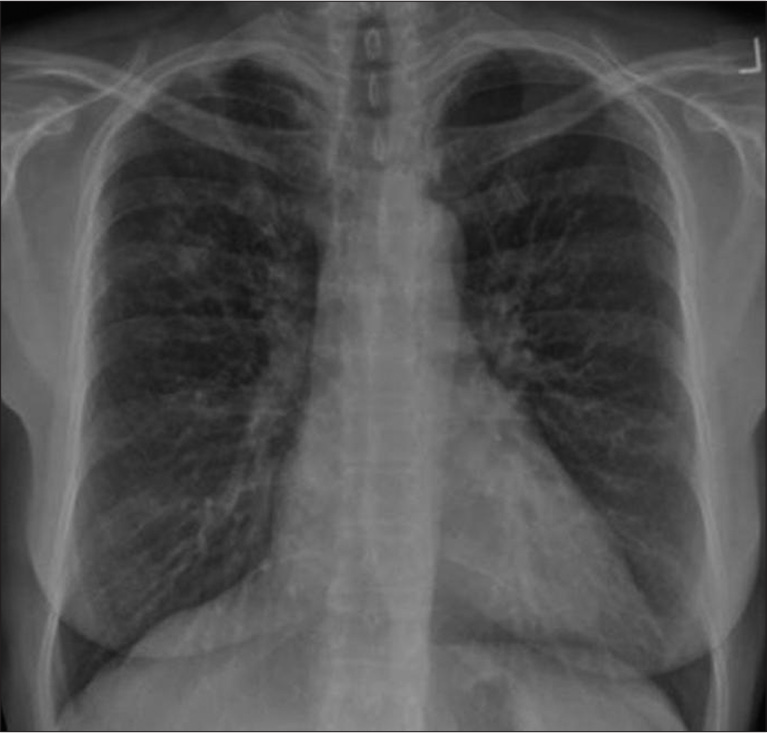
Aspergillosis. ABPA chest X-ray (CXR), manifesting as bilateral consolidation, mostly lower lobe, bronchiectasis and airway impaction or “finger in glove.” The consolidation in the CXR shown is much more diffuse
